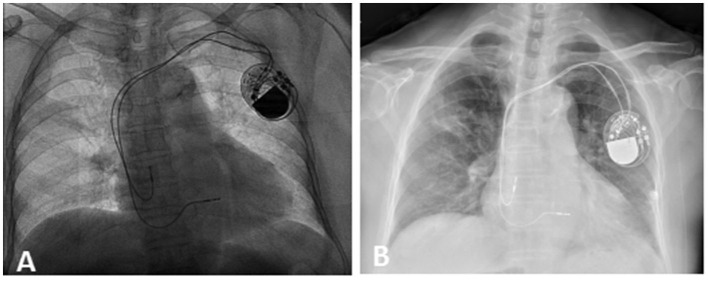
Chest x-ray. X-ray after the first pacemaker operation on September 12, 2019 (A) and on February 16, 2022 (B). This showed correct lead position on admission.
